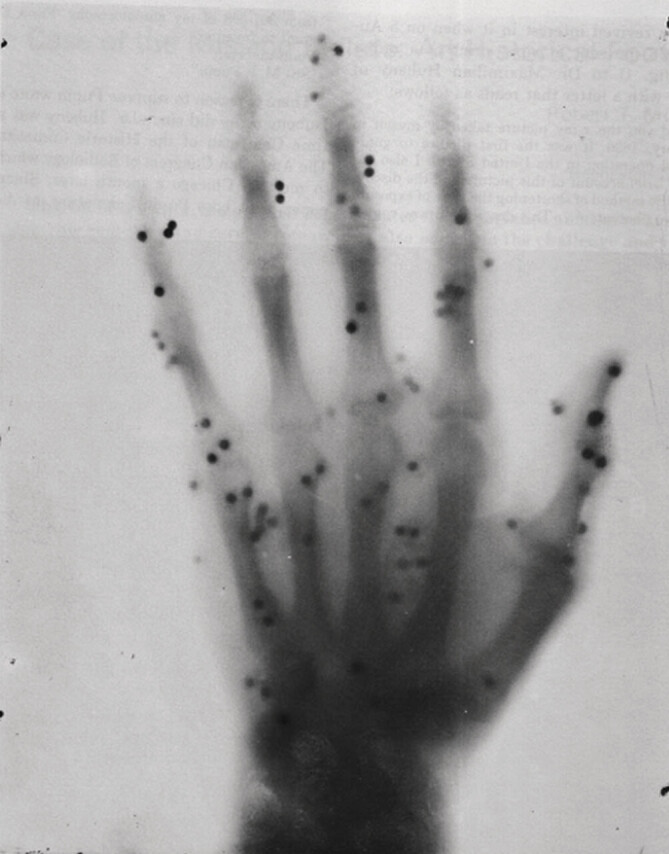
Original photographic plate of the X-ray of Prescott Hall Butler’s left hand containing 77 recognized pellets taken by M.I.Pupin at Columbia College, New York, on February 19,1896. Morrison, M.T. The case of the missing pellets: a historical footnote. Radiology Technology,1974;45(5):328–332 [rerif]
